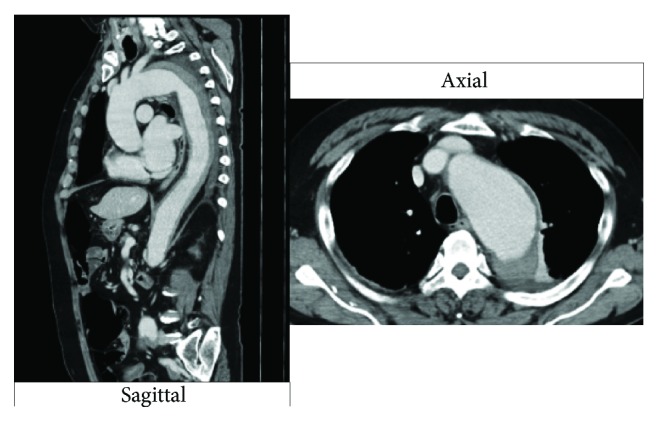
Enhanced CT showing aortic dissection with aortic aneurysm in the descending aorta.
